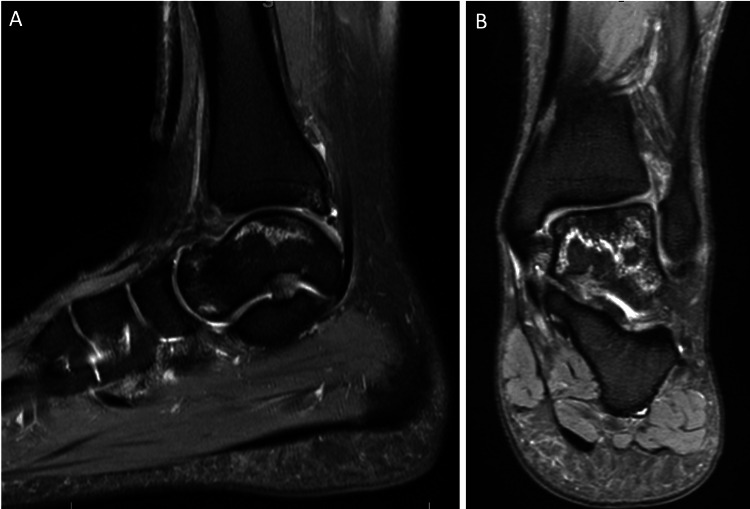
MRI of the left ankle at 12 months from injury - October 4, 2021. (A-B) T2-sequence, sagittal, and coronal views show ischemic bone marrow in a similar pattern with marginal improvement. The posterior tibial plafond re-demonstrates a stable small osteochondral defect.
